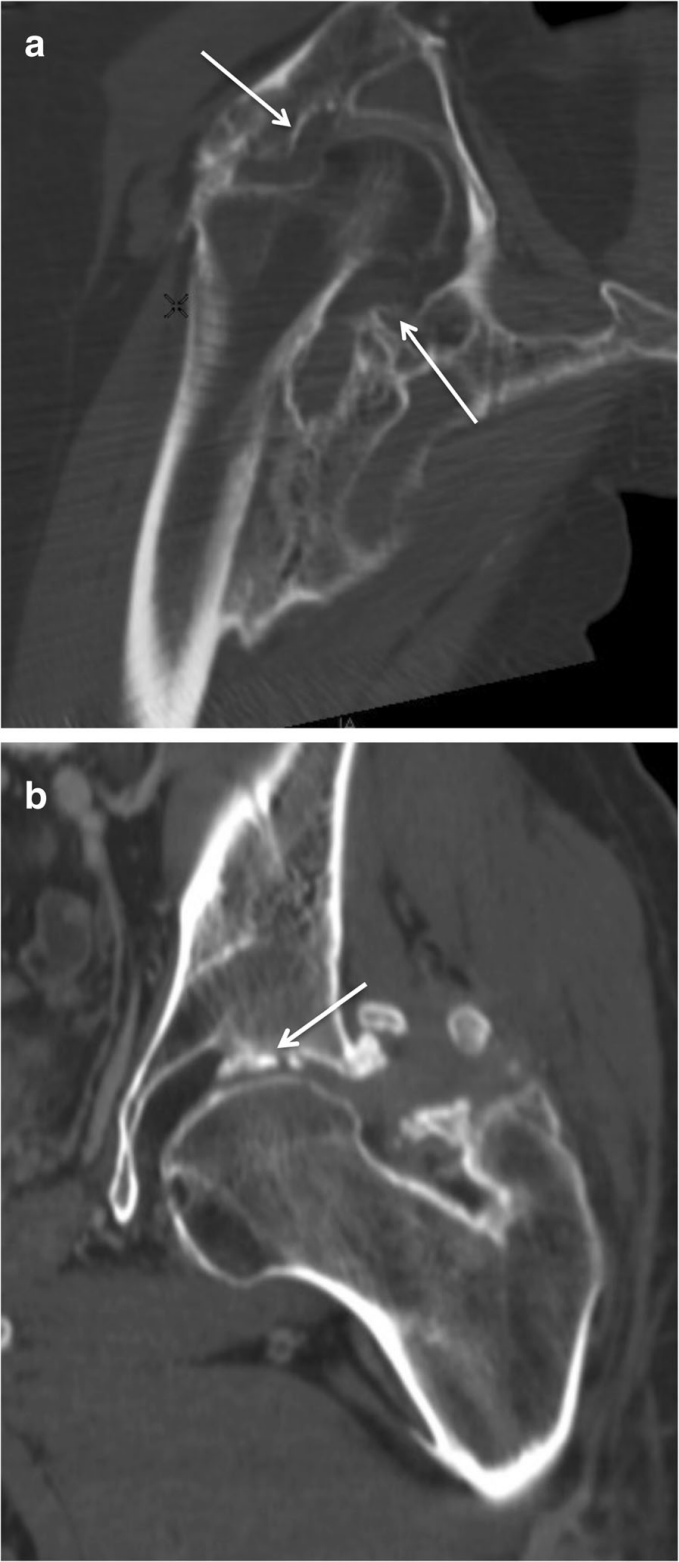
Coronal reconstruction of CT scan: neurogenic osteoarthropathy of the *right* hip (a) and *left* hip (b, other patient). While the osteoma only touches the joint capsule on image A (*arrows*), we can observe a disruption of joint capsule with an involvement of joint space (27 year-old male patient) (*arrow*)
